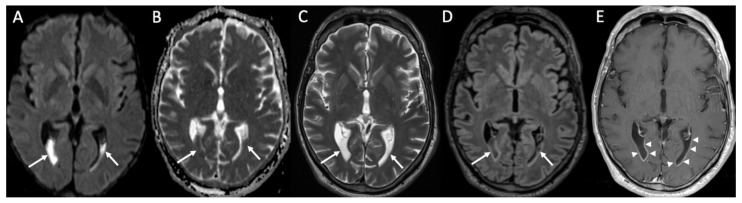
Axial DWI (A), ADC (B), T2 (C), FLAIR (D), and enhanced T1 (E) show purulent material in the lateral ventricles bilaterally (arrows in A–D) with linear subependymal enhancement (arrowheads in E) in a patient with Hemophilus influenzae meningitidis.
